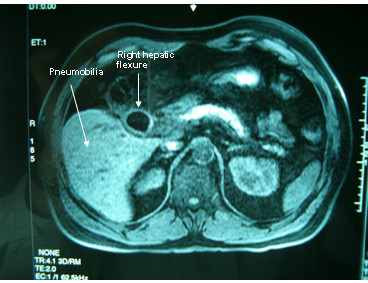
Spiral CT with evidence of pneumobilia and suspicion of cholecystocolic fistula.
